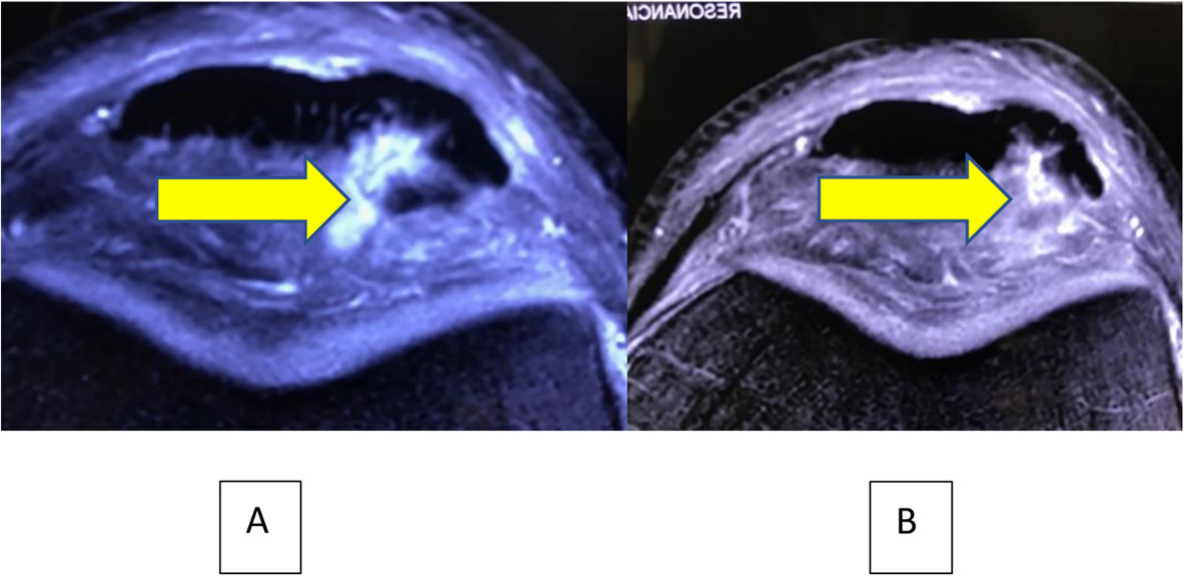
A Pre-injection axial STIR MR image showing injury of the patellar tendon and B: 6 months Post-injections axial view MRI showing partial healing of the tendon. Observe Fat Pad Edema decreasing over a 6 months period. Yellow arrow
